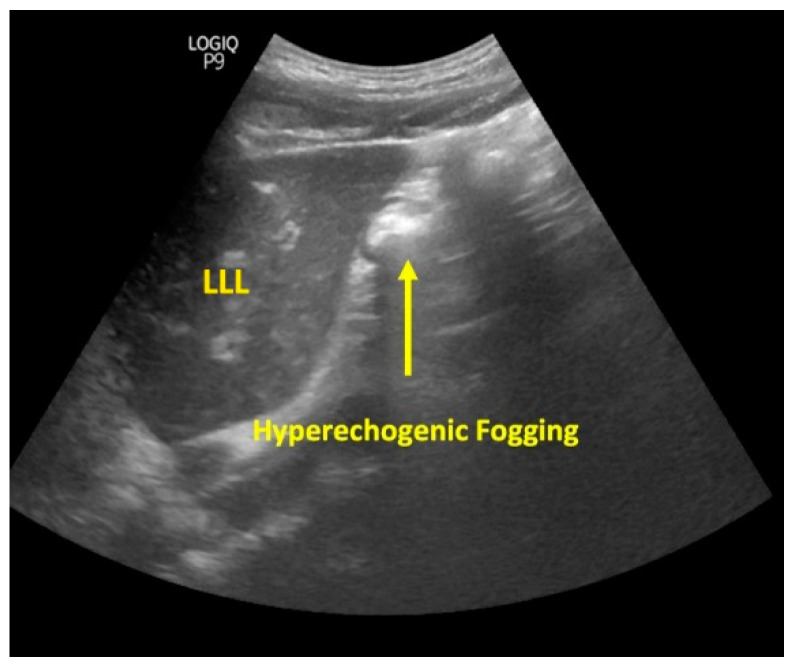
Fogging test ultrasonography image (LLL = left liver lobe).
